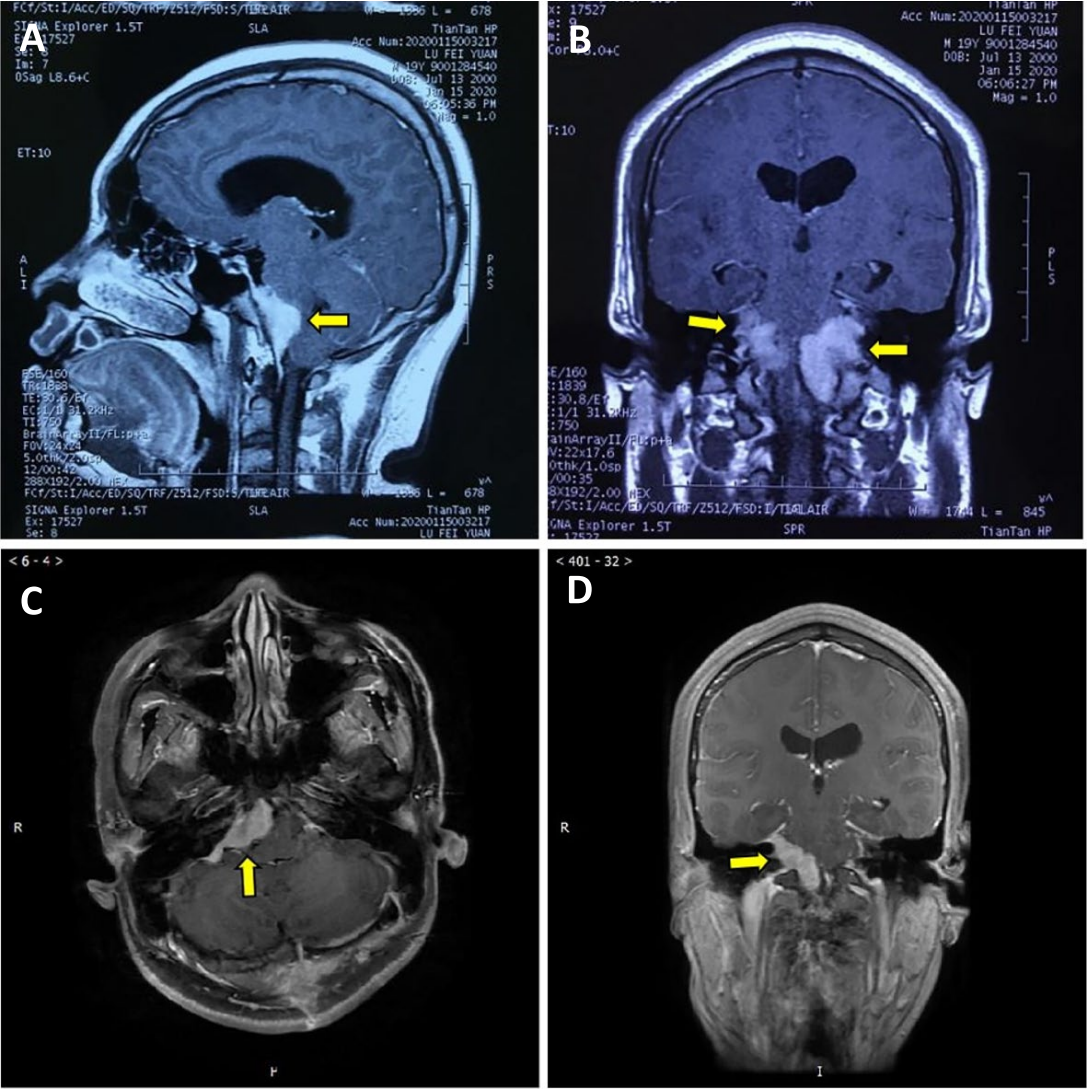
Cranial MRI contrast before and after recurrence. A Enhanced MRI (sagittal view) before recurrence; B Enhanced MRI (coronal view) before recurrence; C Enhanced MRI (transverse sections of the brainstem) after recurrence; D Enhanced MRI (coronal view) after recurrence
